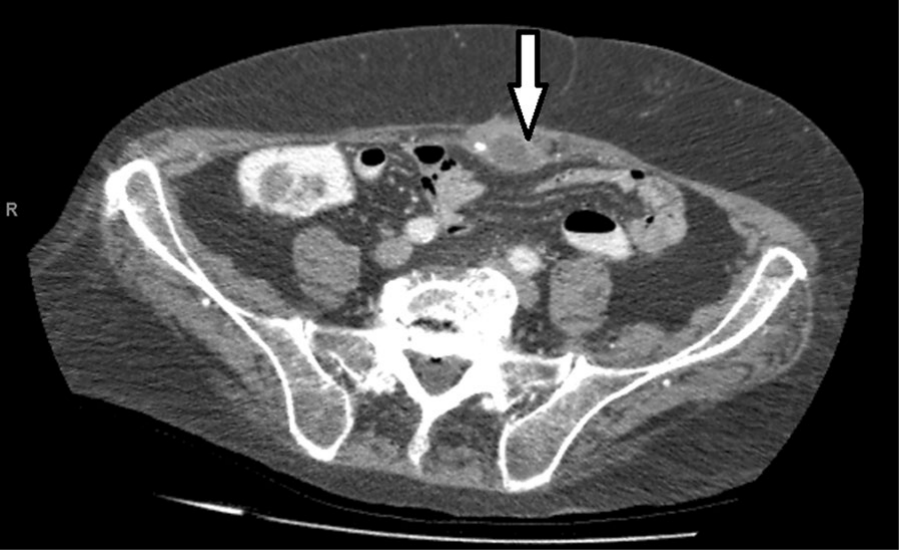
Foreign body on computed tomography scan. The *arrow* highlights the contrast-enhancing abscess cavity next to a calcified foreign body.
